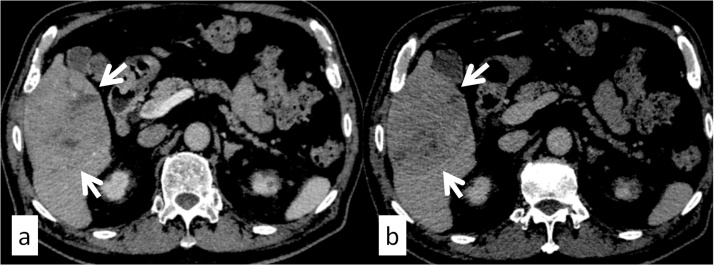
Preoperative computed tomography. A mass in the right lobe of the liver measuring 9.2 × 7.2 cm (white arrow), (a) demonstrating arterial hyperenhancement and (b) washout on delayed images; findings diagnostic of hepatocellular carcinoma.
